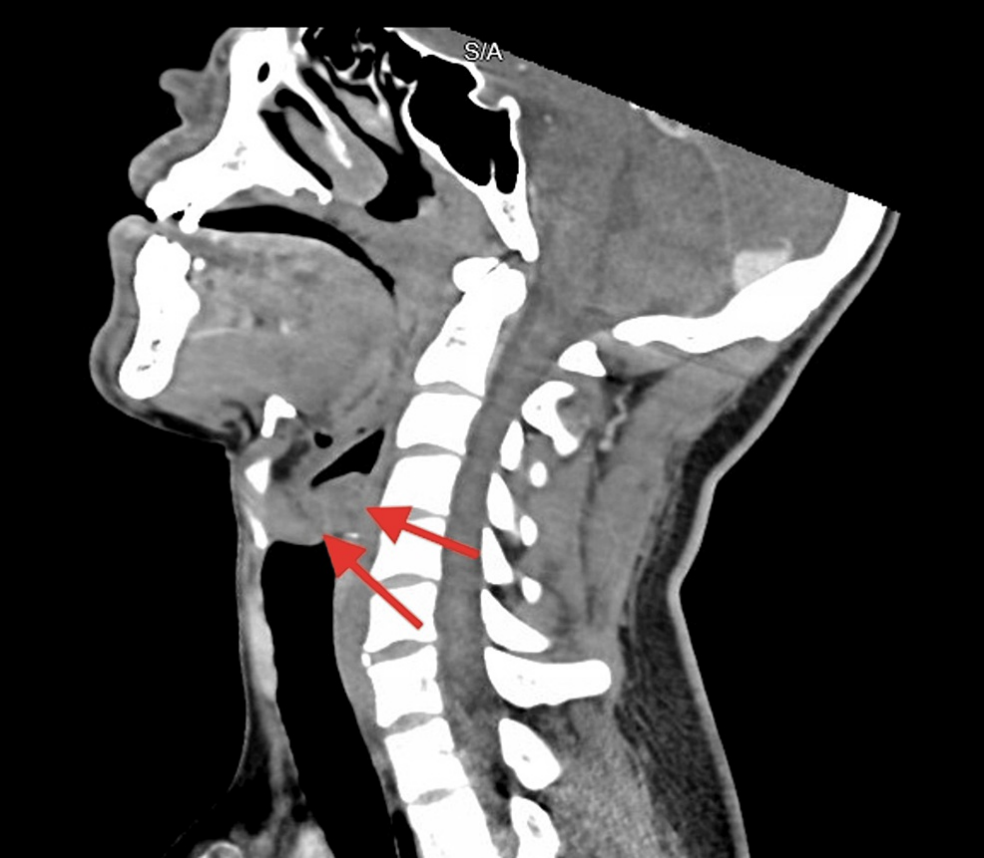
CT of the head and neck in a sagittal view showing mild thickening of the epiglottis (arrows)
